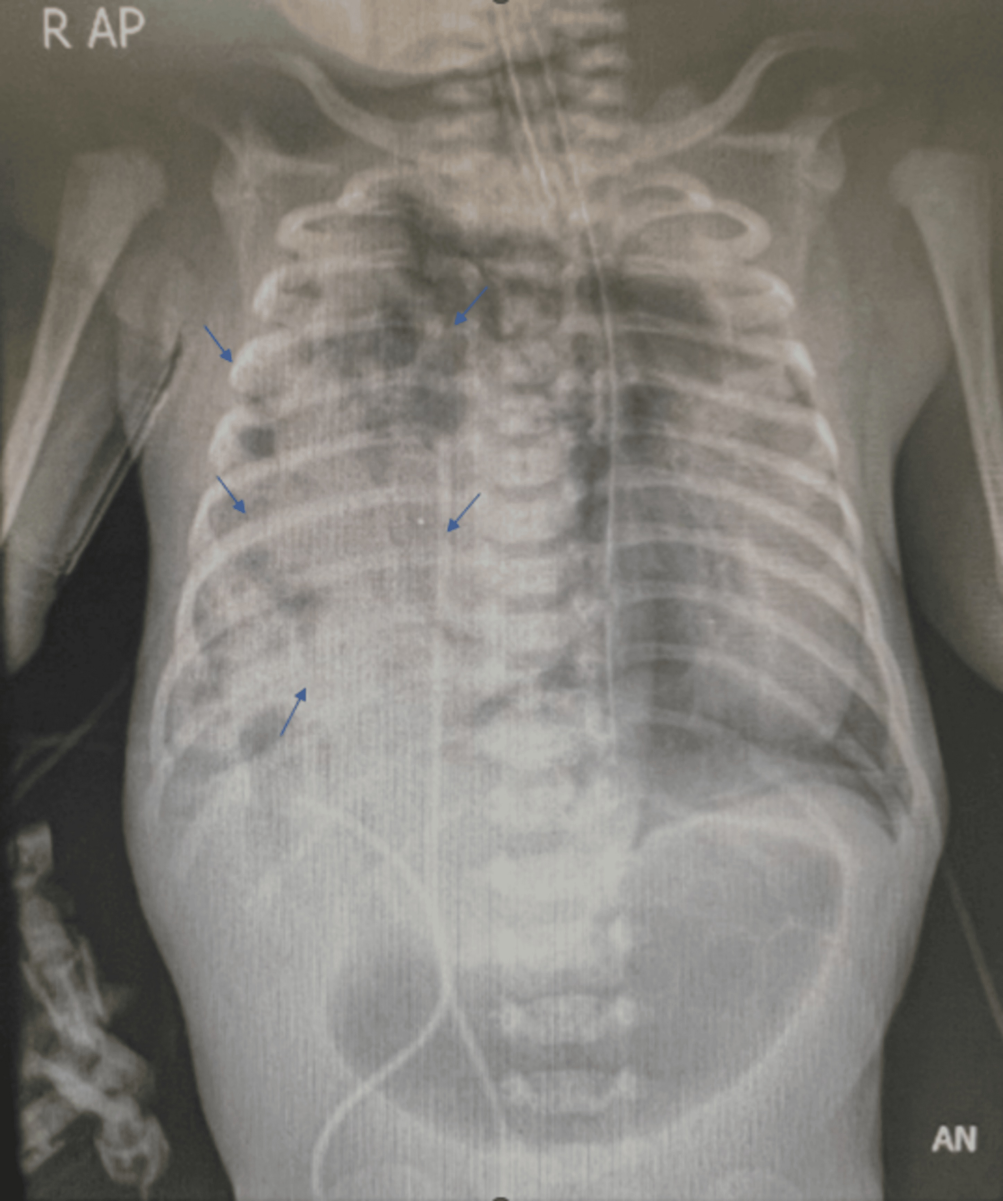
Chest X-ray AP view. Chest X-ray AP view showing the right hemithorax filled with multiple radiolucent regions continuous with the abdominal cavity (arrows), with interval displacement of the mediastinal structures and cardiac silhouette to the opposite side (left). No convincing gas-containing loops of bowel can be seen in the abdomen. These findings implicate multiple loops of bowel occupying the right hemithorax due to the absence of the right hemidiaphragm, resulting from congenital diaphragmatic hernia. AP: Antero-posterior.
